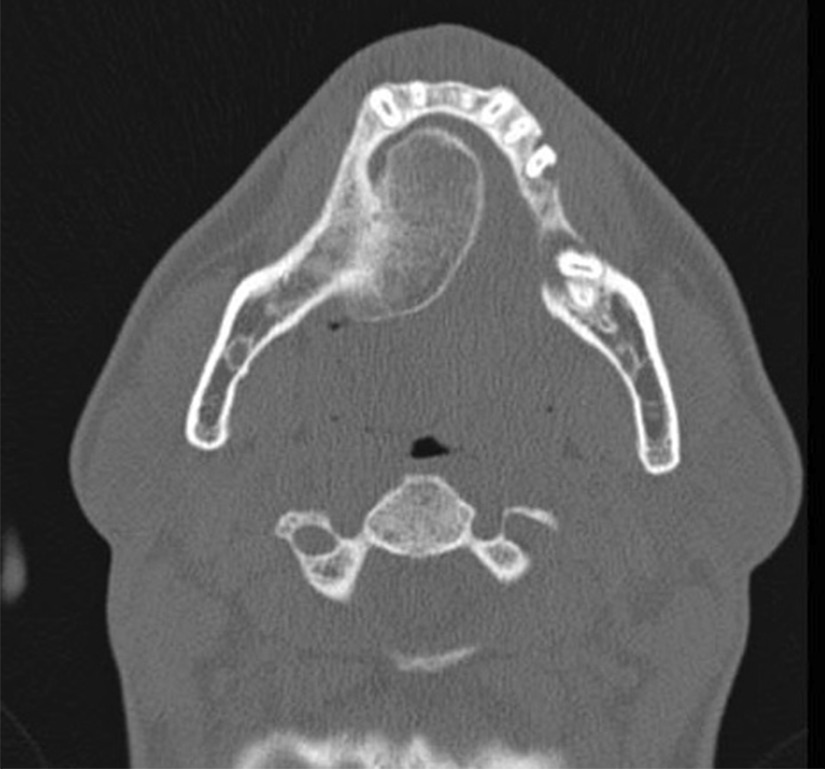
Computed tomography of the lesion in an axial cut, evidencing the mushroom-like shape attached to the alveolar process of the mandible and the radiographic characteristics of a normal bone, with central areal similar to medullary bone, surrounded by thin cortical bone
